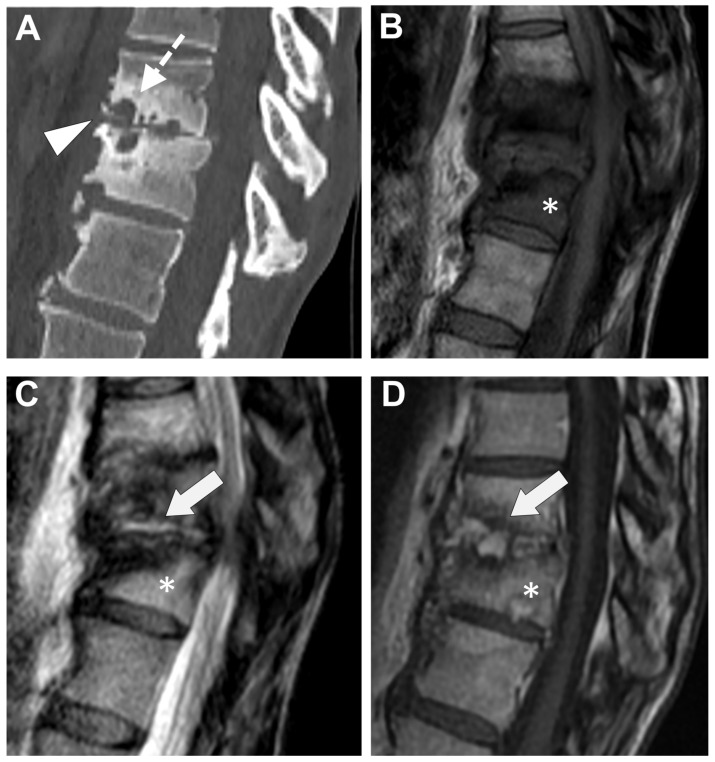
A 67-year-old woman with a medical history of cervical spinal cord injury several years before presented with a rapidly evolving spine deformation at the thoracolumbar junction (kyphosis). On CT (A), there was a strong narrowing of the disc (white arrowhead) with several erosions of endplates from each side (white dashed arrows) and osteosclerosis of the vertebral body. An MRI was performed with (B) T1-weighted imaging (WI), (C) T2-WI, and (D) T1-WI after gadolinium chelates injection. This examination demonstrates abnormal signal intensities (SIs) of the disk with SIs (fluid-like) on T2-WI, with a contrast enhancement (white arrows). The vertebral bodies showed an edema with low SIs on T1-WI, high SI on T2-WI, and contrast enhancement (asterisk).
